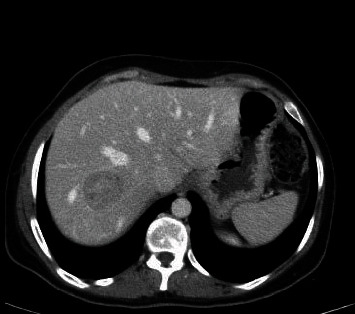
Axial CT view of the lesion, showing the lesion invading the liver parenchyma.
